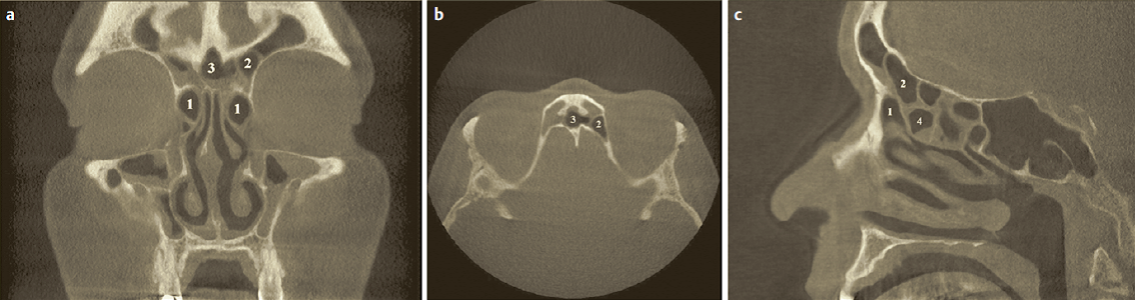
Frontal sinus drainage type I according to Draf = complete resection of the uncinate process and resection of parts of the medial lamella of the agger nasi cell and the anterior wall of the ethmoid bulla if needed [242, 246, 248, 359]. A different postoperative situation results depending on the individual anatomy: on the right isolated agger nasi cell, on the left side additional posterior frontoethmoidal cell (frontal bulla), intersinus septal cell; 1 = agger nasi cell, 2 = posterior frontoethmoidal cell (frontal bulla), 3 = interfrontal sinus septal cell, 4 = ethmoid bulla; a) coronal CT scan, b) axial CT scan, c) sagittal CT scan.
